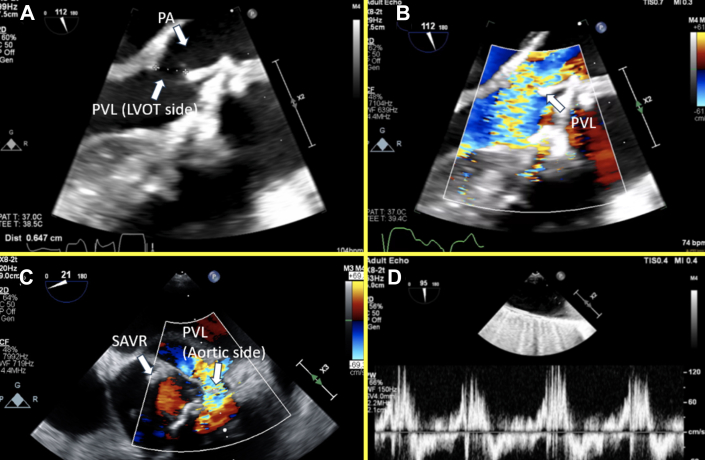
Transesophageal Echocardiography of Paravalvular Leak (A) Transesophageal echocardiography shows PVL originating from a pseudoaneurysm into the LVOT. (B) Color Doppler reveals severe regurgitation through the PVL. (C) The PVL originated above the surgical aortic valve (on the aortic side), and flow wrapped around a serpiginous tract. (D) There was evidence of severe aortic insufficiency, such as holodiastolic reversal. LVOT = left ventricular outflow tract; PVL = paravalvular leak; SAVR = surgical aortic valve.
